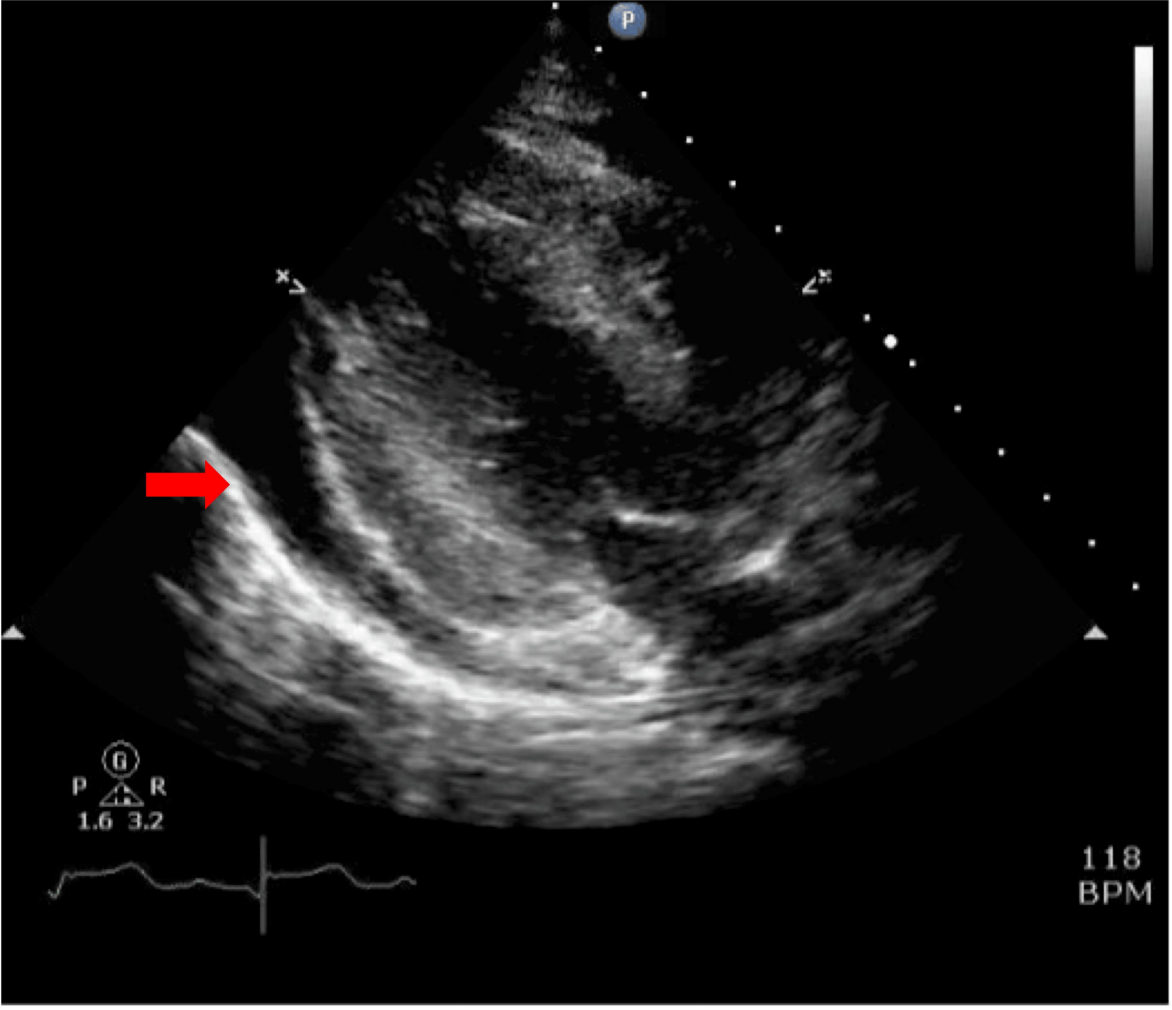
Echocardiography on ICU day 2 Transthoracic echocardiography was performed on ICU day 2, several hours before pericardial drainage. The arrow indicates pericardial effusion.
